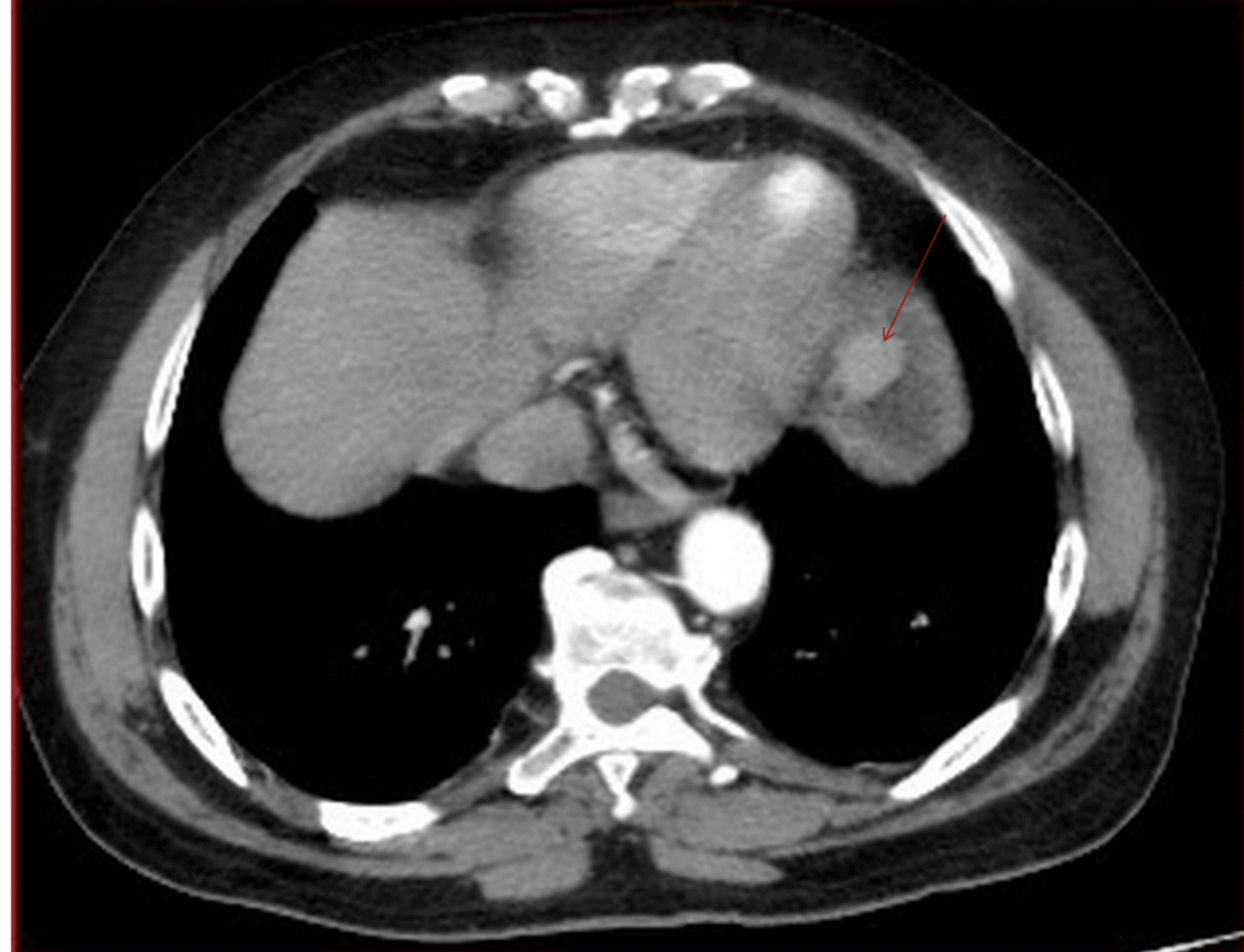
Contrast-enhanced CT scan of the chest and abdomen showing a gastric fundal mass A well-defined, rounded mass measuring 2.4 cm X 1.8 cm with homogeneous moderate enhancement is located at the gastric fundus. The mass shows an endoluminal growth pattern.
